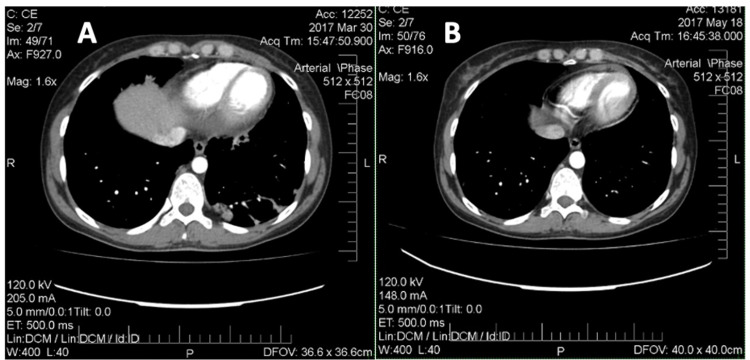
Multislice computed tomography of the chest (2017). A: Signs of bilateral focal pneumonia, multiple infiltrates (March 2017). B: The reverse development of foci in the lungs (May 2017).
